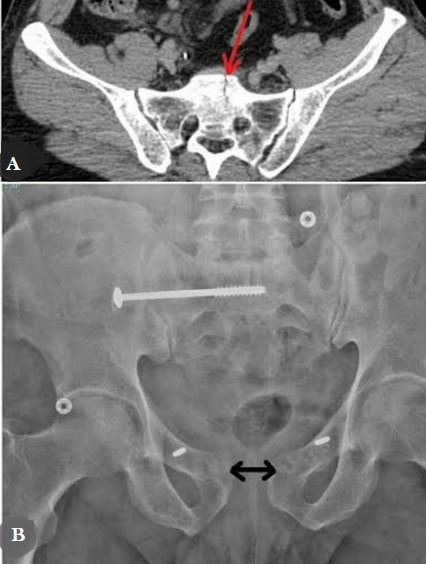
A) scanner du bassin montrant la fracture du sacrum; B) radiographie de face du bassin après 6 mois montrant la bonne consolidation du sacrum et le petit distasis au niveau de la symphyse pubienne
